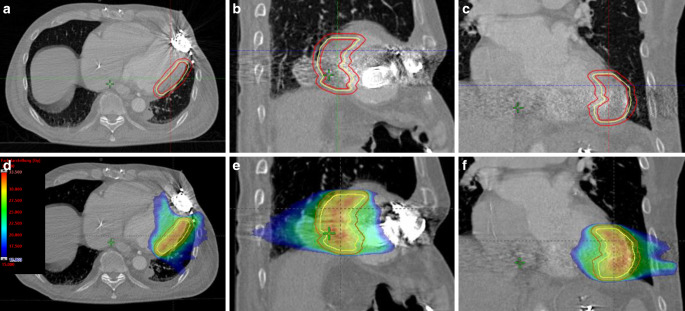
Case II: clinical (*inner red contour*) and planning target volumes (*larger red contour*) and integrated target volume (*yellow line*) in axial, sagittal, and coronal views (a–c). Dose distribution of radiosurgery treatment plan in corresponding levels of planning computed tomography (d–f) together with a dose scale (4d). Dose distribution is displayed to 15 Gy; ITV and PTV contours are displayed
